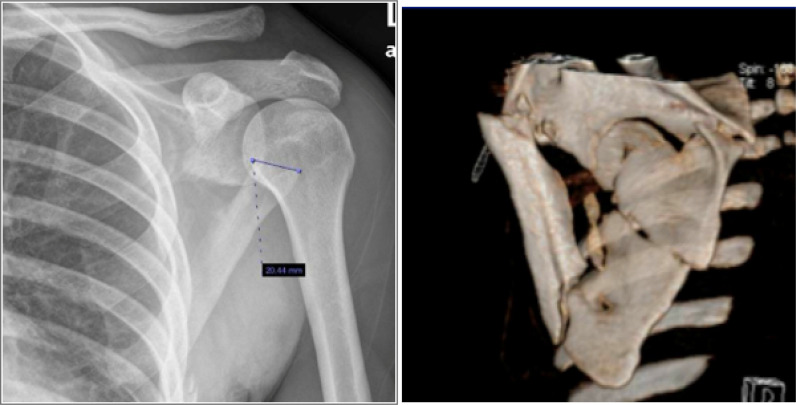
Anteroposterior shoulder radiograph and three-dimensional reconstruction image of a patient with extra-articular fracture that was treated nonoperatively.
